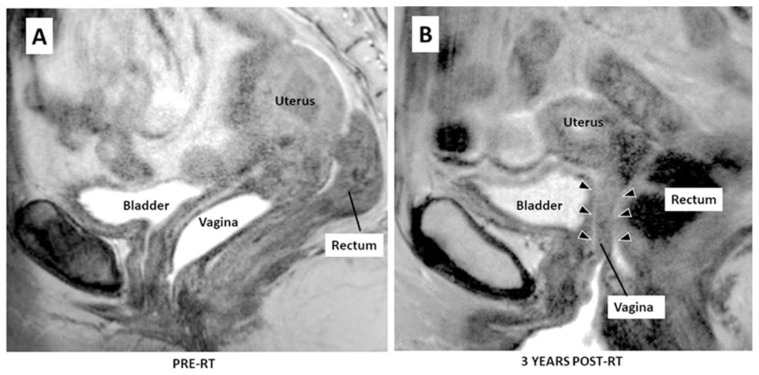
MRI image (A) before and (B) after pelvic irradiation treatment. The black arrows of (B) show the shorting and narrowing of the upper 2/3 of the vaginal anatomy [14].
